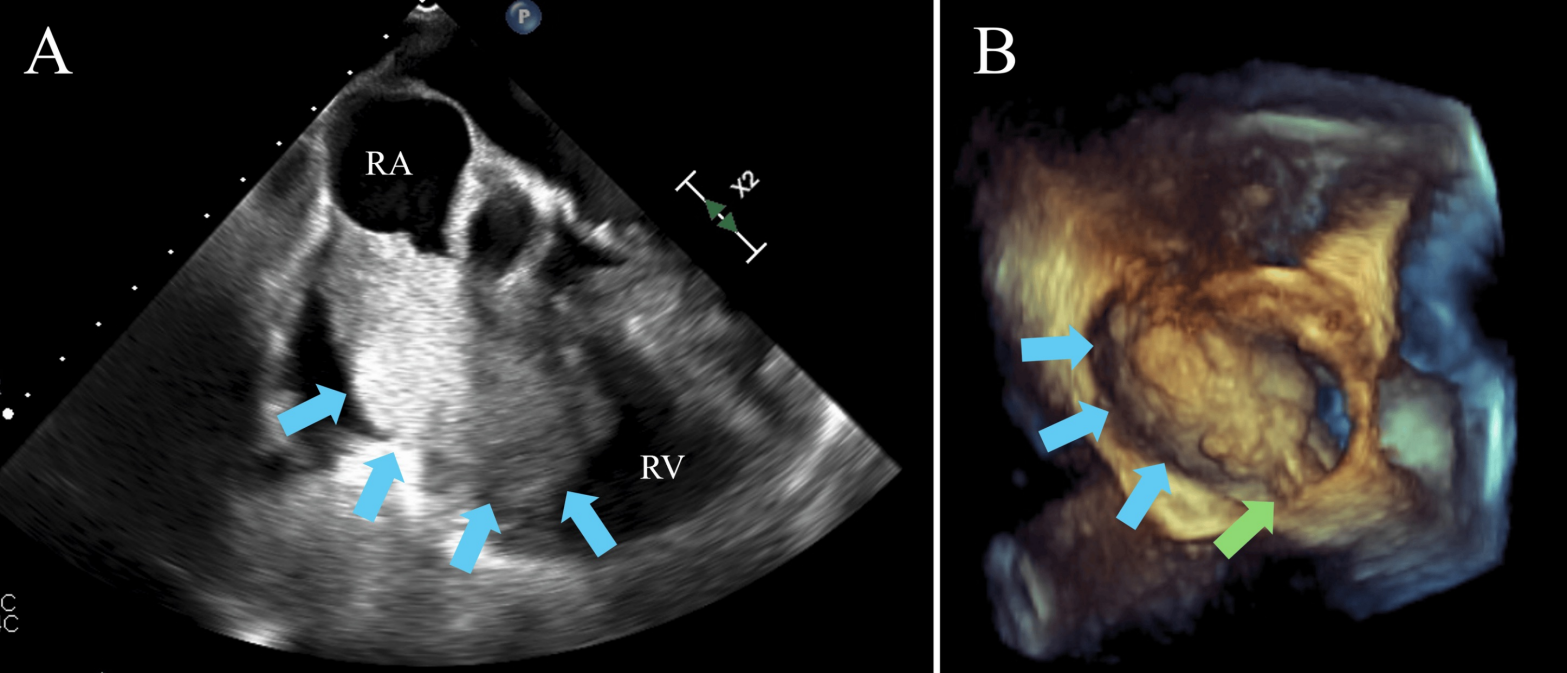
Transesophageal echocardiography images. (A) The tumor nearly prolapsing toward the tricuspid valve. (B) Three-dimensional reconstructed image showing a pedunculated tumor originating from within the right atrium. Blue arrow: tumor; green arrow: pedicle.
